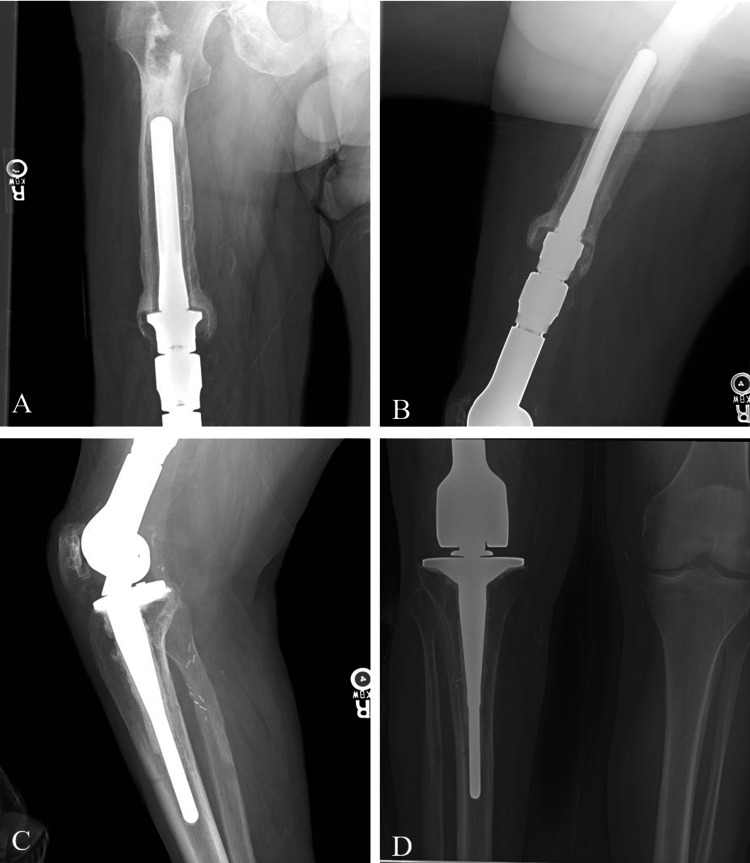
Pre-operative anterior-posterior (AP) x-rays of the thigh (A) and knee (D) as well as lateral x-rays of the thigh (B) and knee (C).
